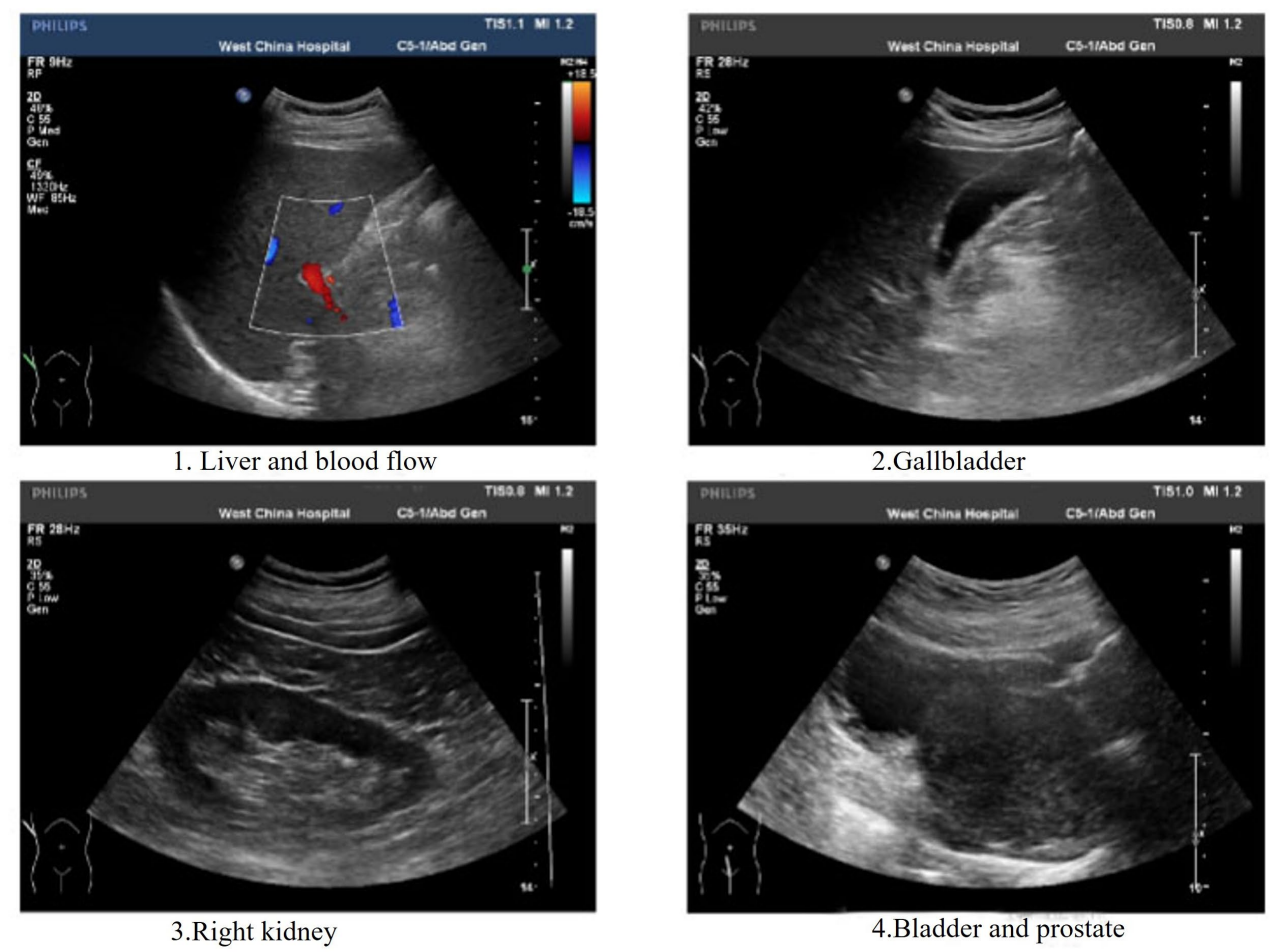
As observed in the color ultrasound images: Picture 1 shows a normal liver morphology, characterized by homogeneous parenchymal echogenicity and the absence of occupying lesions. In Picture 2, the gallbladder appears normal in size, with several slightly echogenic attachments on the wall, the largest measuring approximately 0.5 cm. No abnormal echoes are detected within the cystic cavity. Picture 3 reveals a strong echogenic mass of about 0.5 cm in the lower pole of the right kidney, with no distinct hypoechoic areas in the collecting system. Moving to Picture 4, the anteroposterior diameter of the prostate is approximately 5.3 cm, protruding into the bladder by about 1.8 cm, and displaying heterogeneous parenchymal echogenicity.
